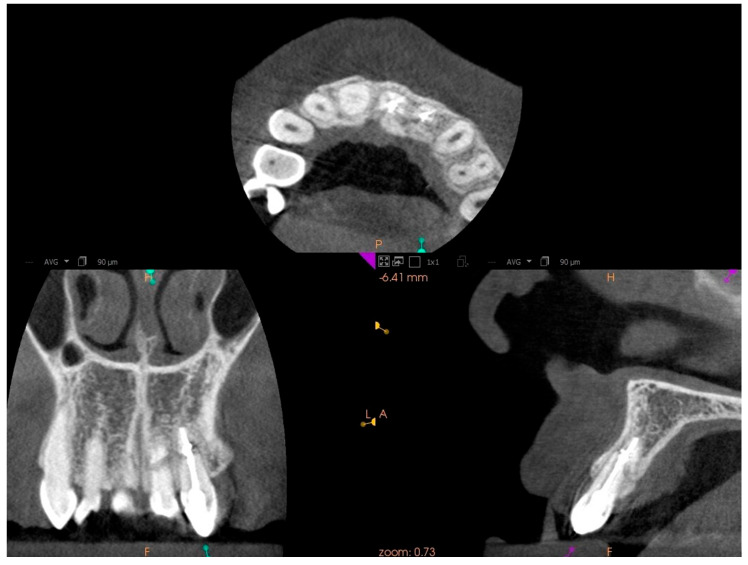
CBCT images presenting tooth no. 22 after 12 months of treatment.
